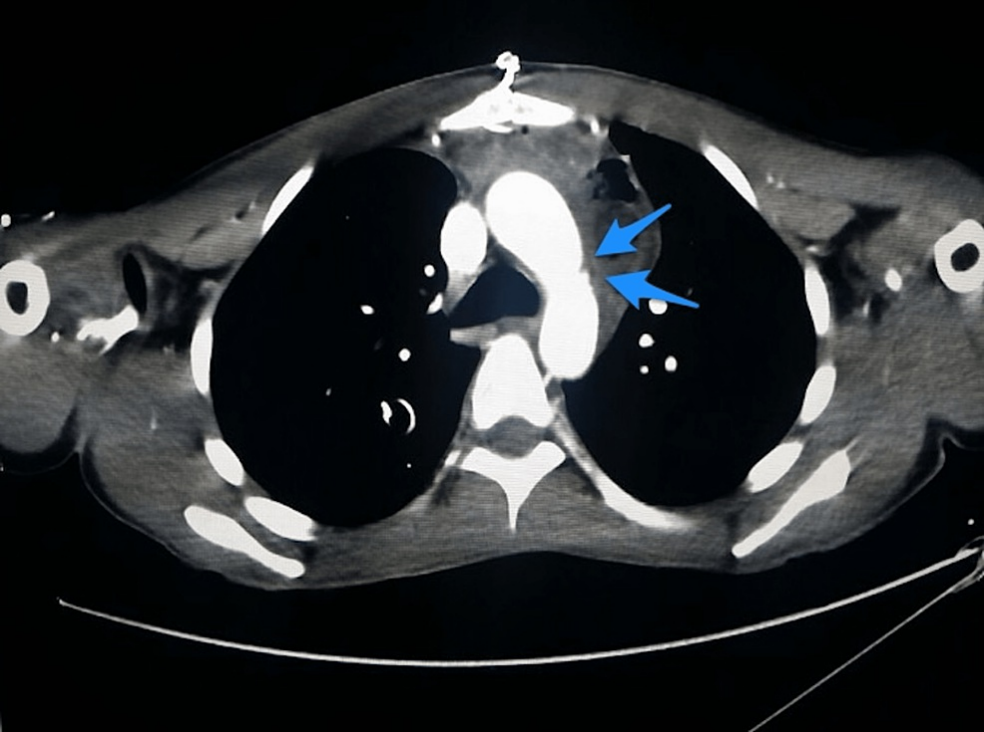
Follow-up axial CT angiography demonstrates no contrast extravasation with irregularity in the wall (arrows).
